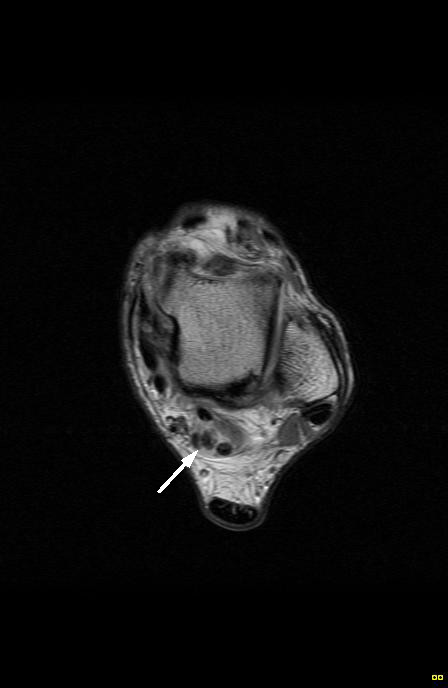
Axial MRI of the left ankle (proton density) demonstrates (arrow) multiple heterogeneous and low signal intensity nodules within the extended flexor hallucis longus tendon sheath.
